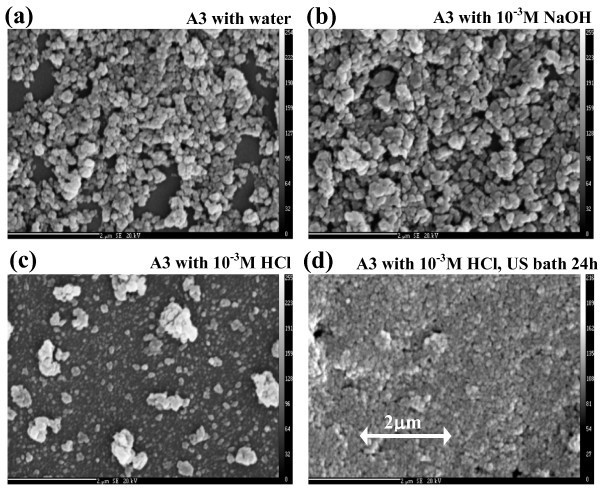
Examples of SEM images of dried samples. The representative photographs were selected for each tested sample. In contrast to the samples (a, b), the samples (c, d) contain a major part of the nanopowder in the form of fine particles. In addition, the long-time ultrasonification, see sample (d), breaks-up the remaining clusters apparent in sample (c). The specification of A3 nanopowder is given in Table 1.
